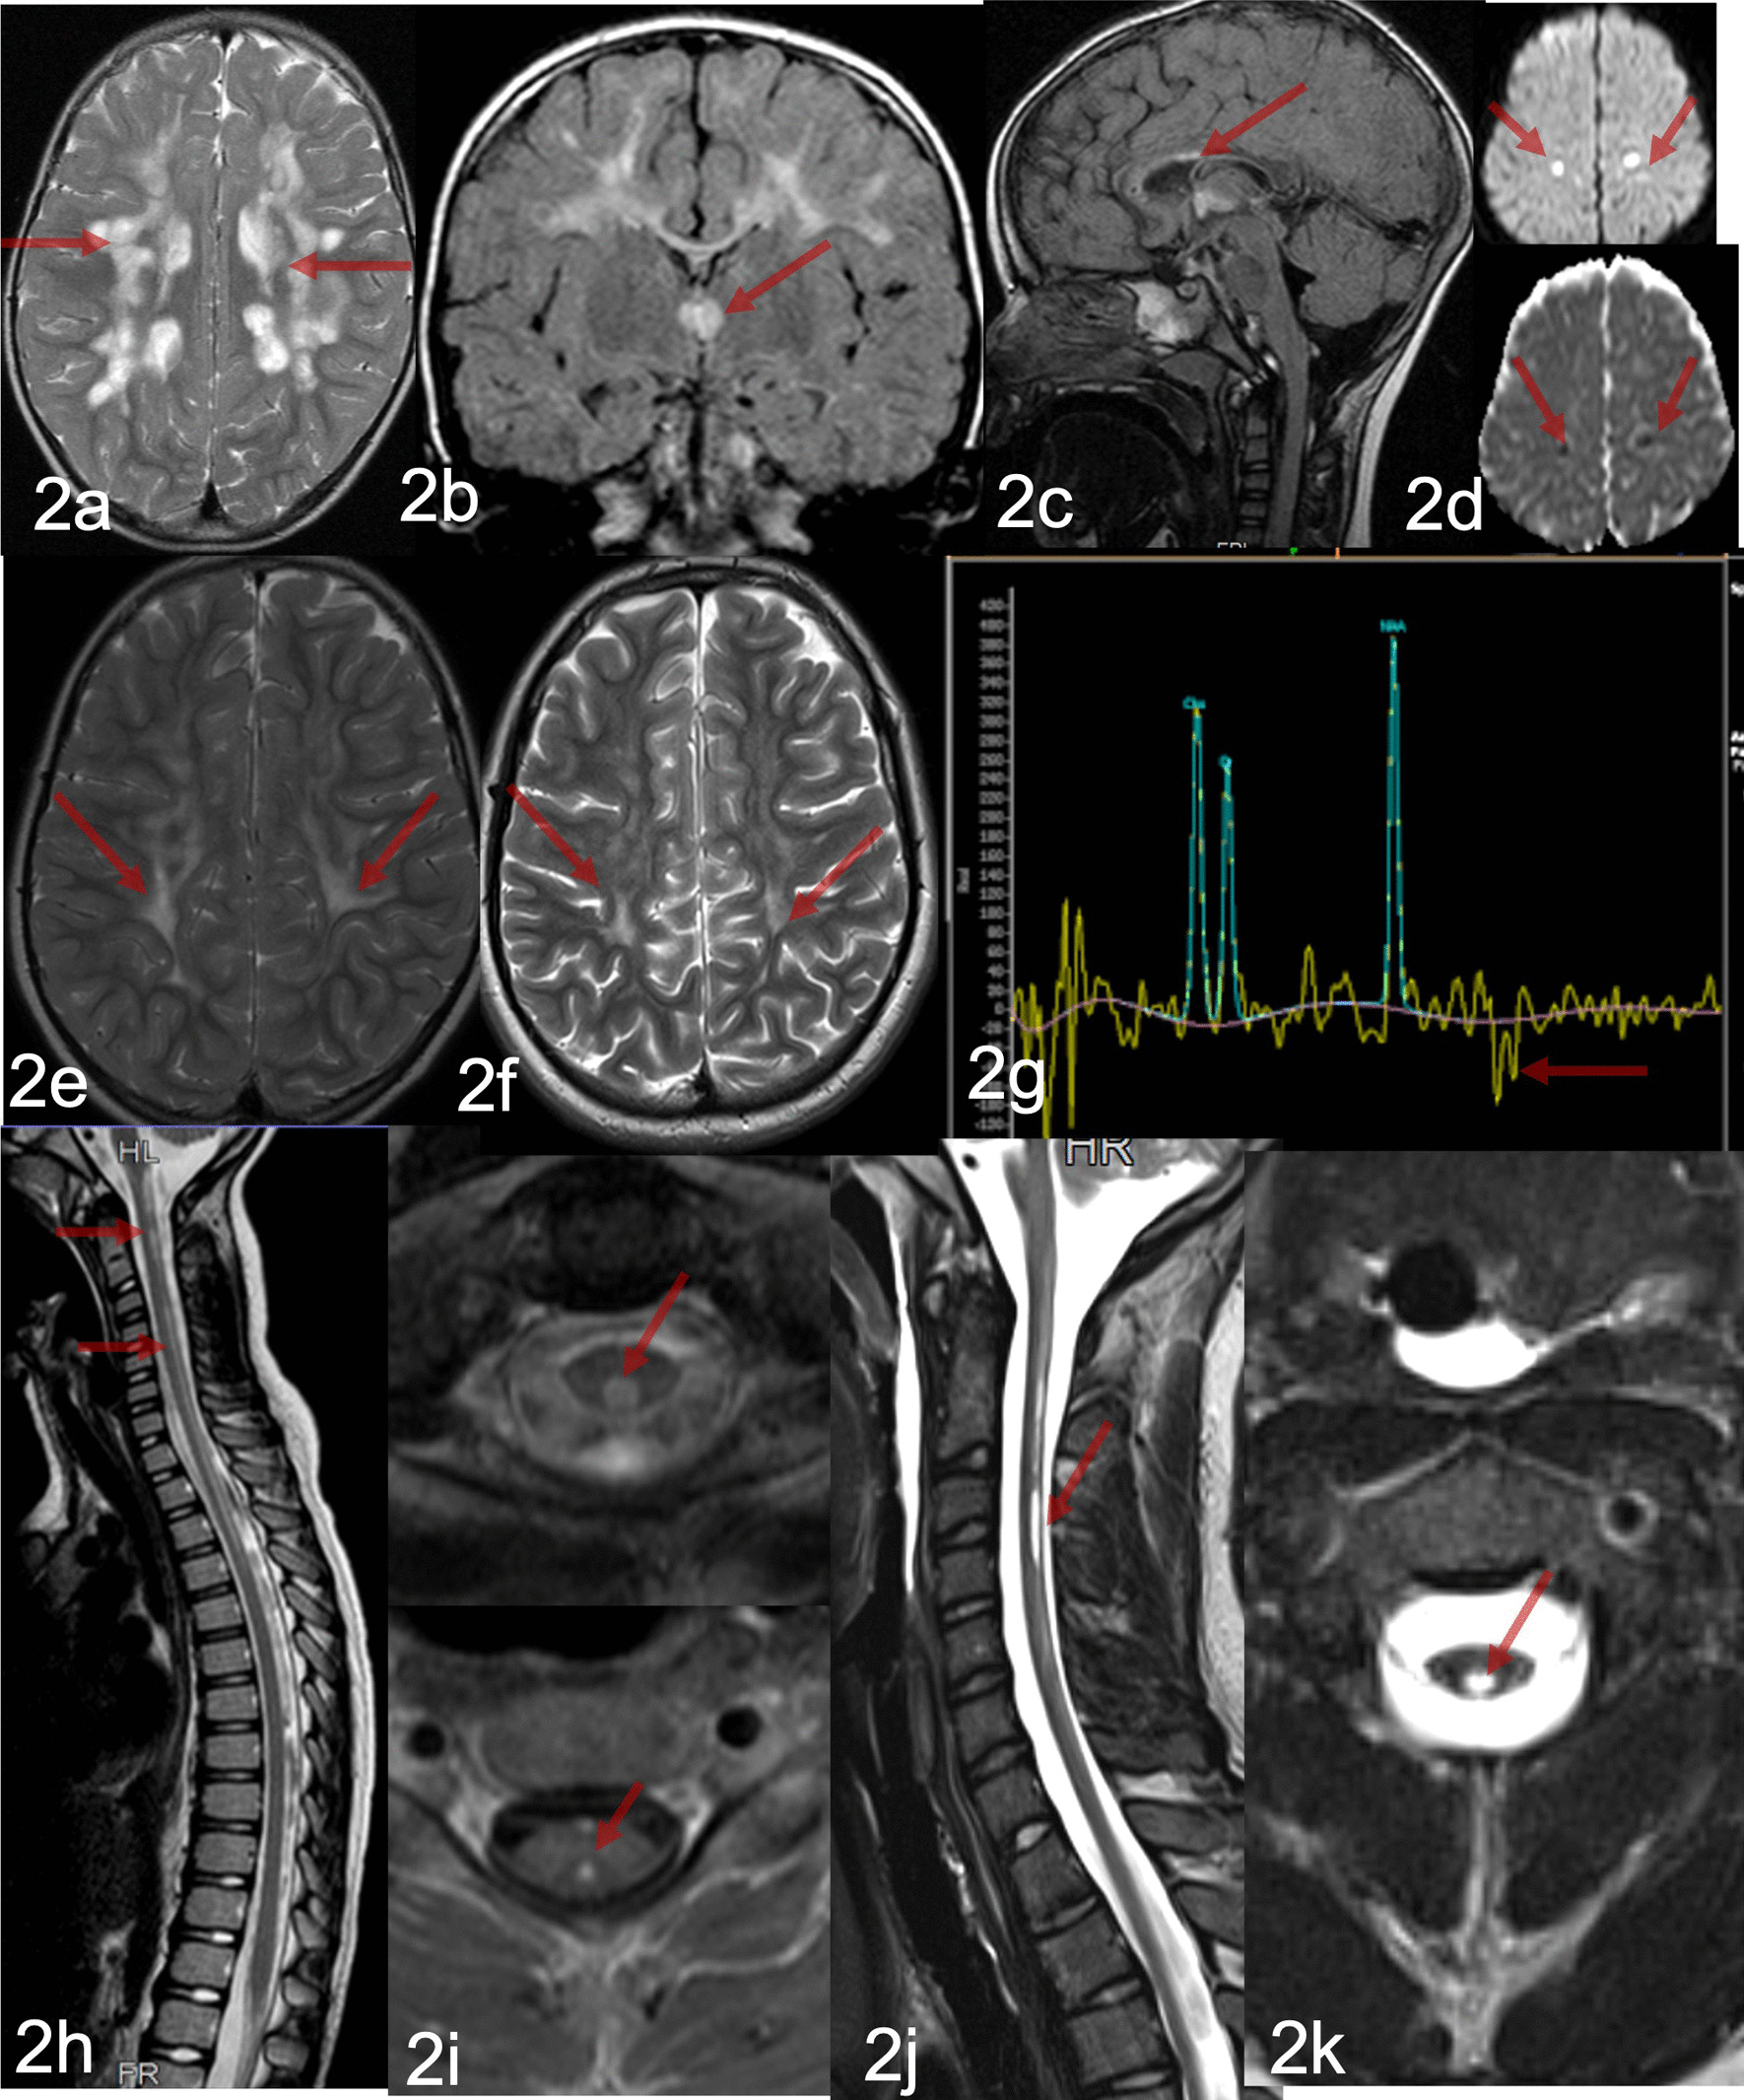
Serial MRI Brain and Spine in Patient 1. Brain magnetic resonance imaging (MRI) at the age of 2½ years (a—T2 weighted axial view) shows hyperintense confluent signal changes in the periventricular and deep white matter (a). MRI obtained 10 months after presentation showed additional lesions in thalamus and corpus callosum (b, c arrows). Diffusion-weighted imaging (d upper panel and corresponding ADC maps, Fig 1d lower panel) showed restricted diffusion of few white matter lesions (arrows)). e T2 weighted axial view: At the age of 5 years MRI showed attenuation of the white matter lesions MR examination at the age of 17 years the white matter lesions were unchanged (f, T2 weighted axial). g MRS showed lactate peak on multiple occasions. h T2w sagittal: MRI spine at the age of 5 years shows spinal cord lesions in the dorsal and central regions extending from the cervico-medullary junction down to T4). The spinal cord lesions showed enhancement with contrast (i—T1 w axial image, upper panel, and (lower panel, contrast enhanced image). The spinal cord lesions showed gliotic changes (j, T2 weigted sagittal and k, T2 weighted axial)
